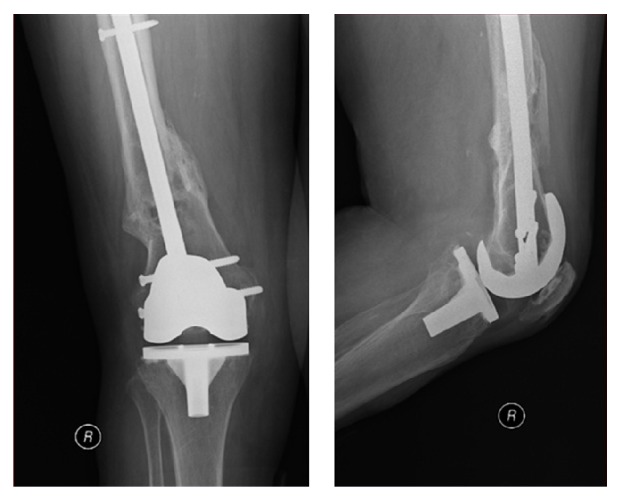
24 months of postoperative AP and lateral right knee X-rays showing complete consolidation of the fracture.
